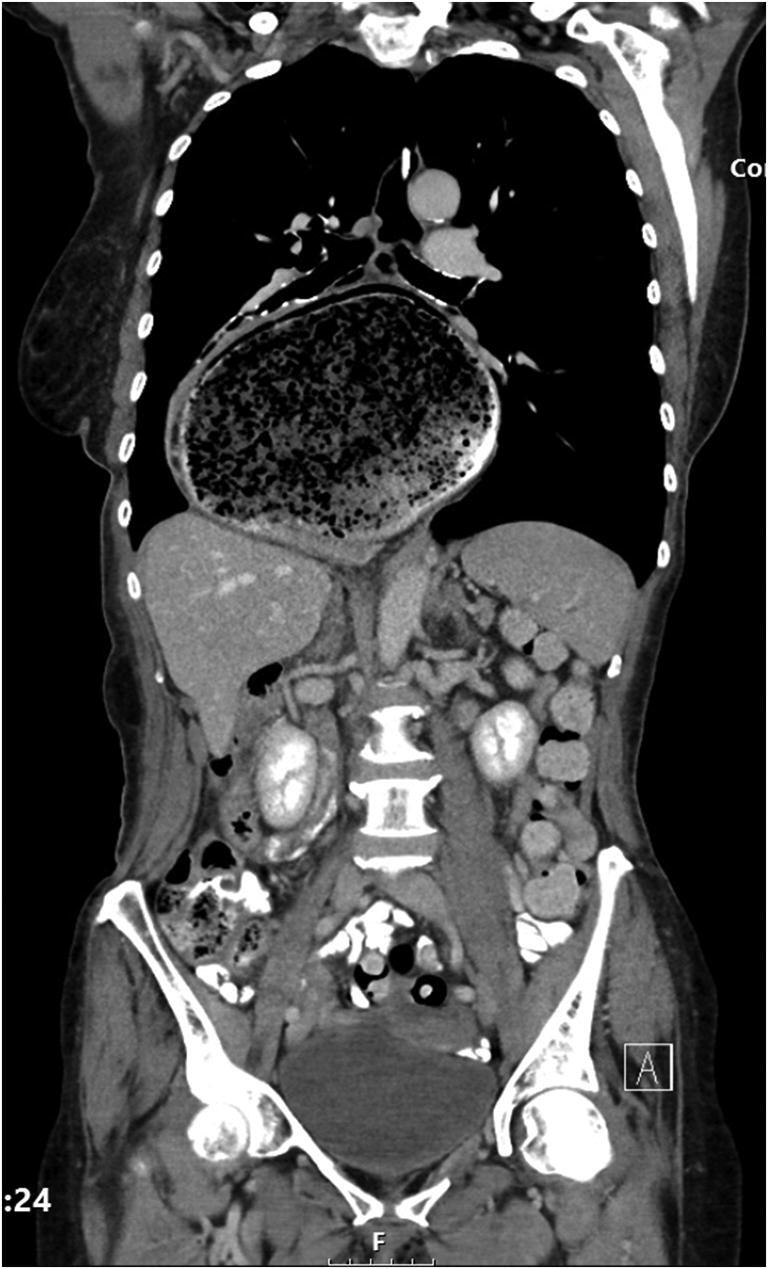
CT scan showing food in the giant hiatus hernia in the chest
